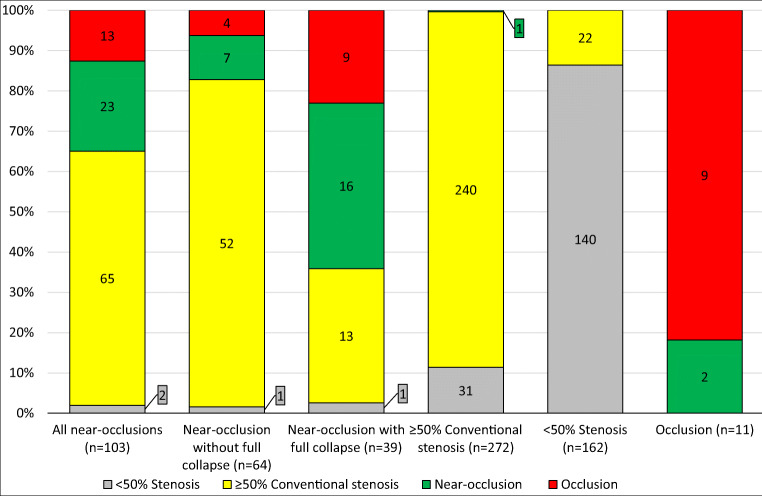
Comparison of CT-angiography (bars) and ultrasound findings (colors). Near-occlusion on ultrasound denotes *any indication of near-occlusion*, where two cases with <50% stenosis had *intermediate PSV* and diagnosed as <50% stenosis in the report
